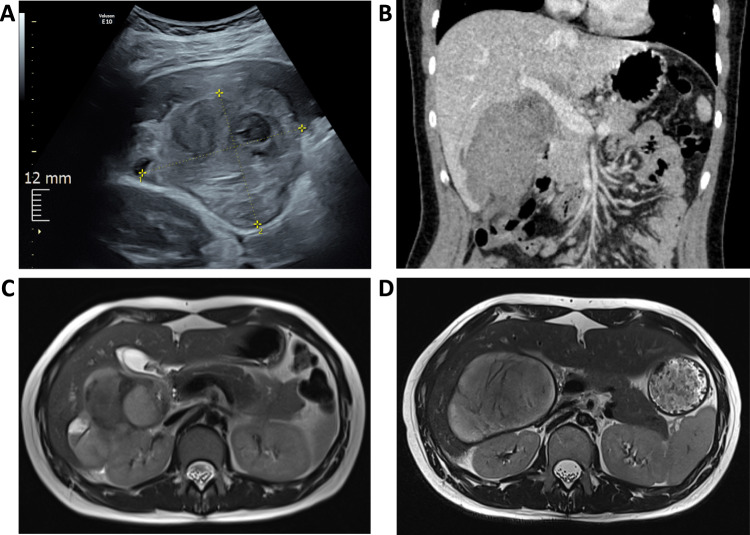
Imaging studies of the 24-year-old patient presenting with hepatic pregnancy. A Emergency transabdominal ultrasound on primary presentation showed a 9 × 5 × 4 cm inhomogeneously hyperechogenic tumor with hypoechogenic circumscribed areas on the right hepatic lobe. B Contrast-enhanced abdominal CT, coronal plane, exhibiting an intermediate- to low-density tumor measuring approximately 10 × 7 × 11 cm, attached to the right hepatic lobe with hilar involvement. C 1.5-Tesla-MRI, T2-HASTE, axial plane, displaying an 11 × 6 cm lobulated inhomogeneous tumor with solid and liquid components located in the Morison pouch. D 3-Tesla-MRI, T2-HASTE, axial plane, shows an increasingly circumscribed, roundish tumor with intermediate T2 internal signal, T2 hypointense encapsulation and septa-like central parts. The tumor has remained stable in size
